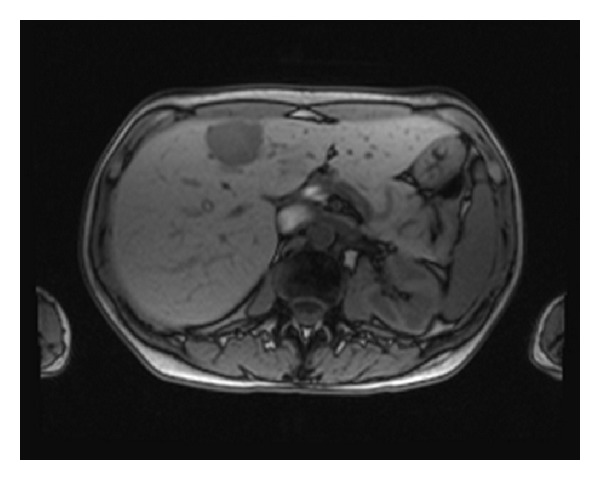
T1 hypointense liver lesion on MRI.
